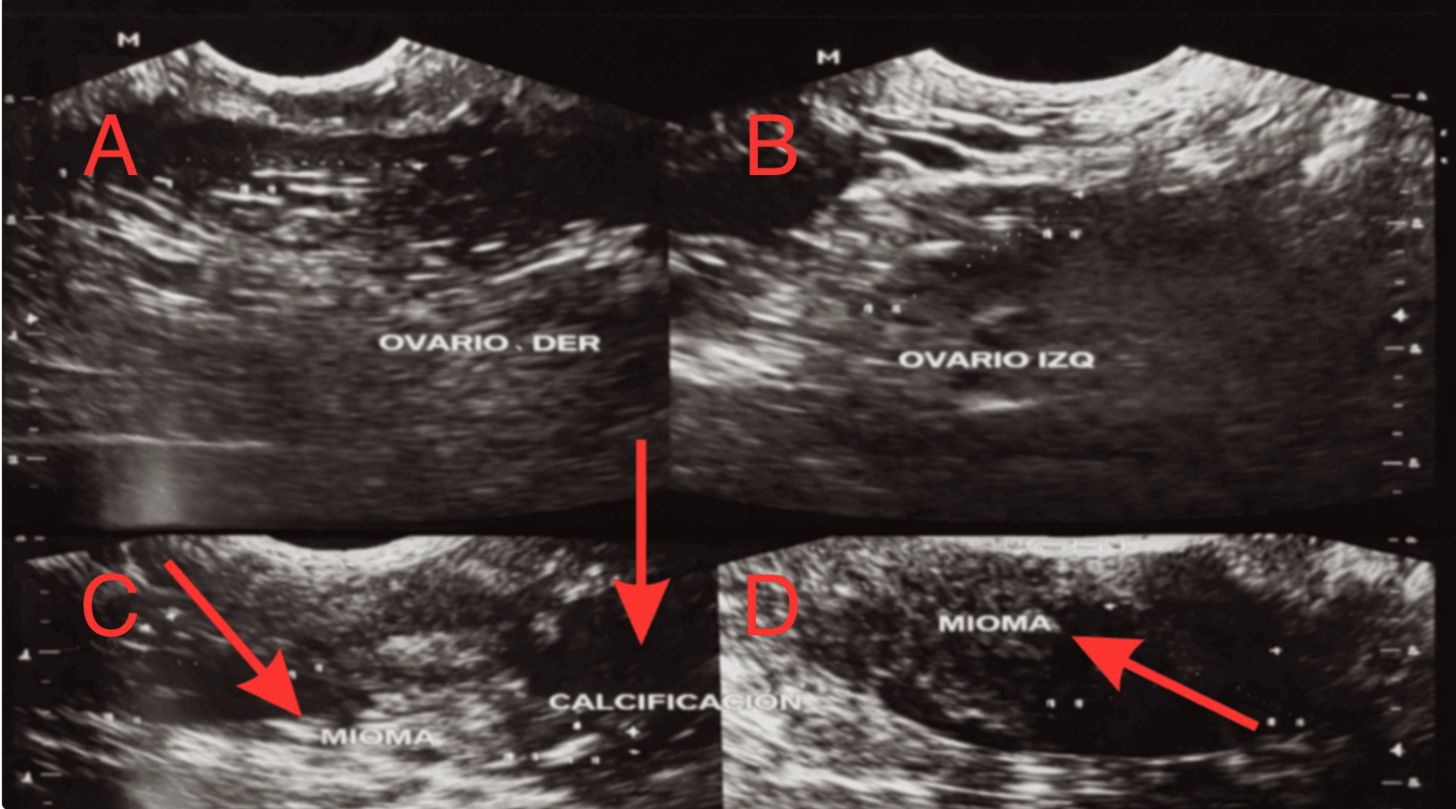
Transvaginal ultrasound findings of the uterus and adnexa (left to right). (A) Right ovary with normal size and echotexture, without focal masses.
